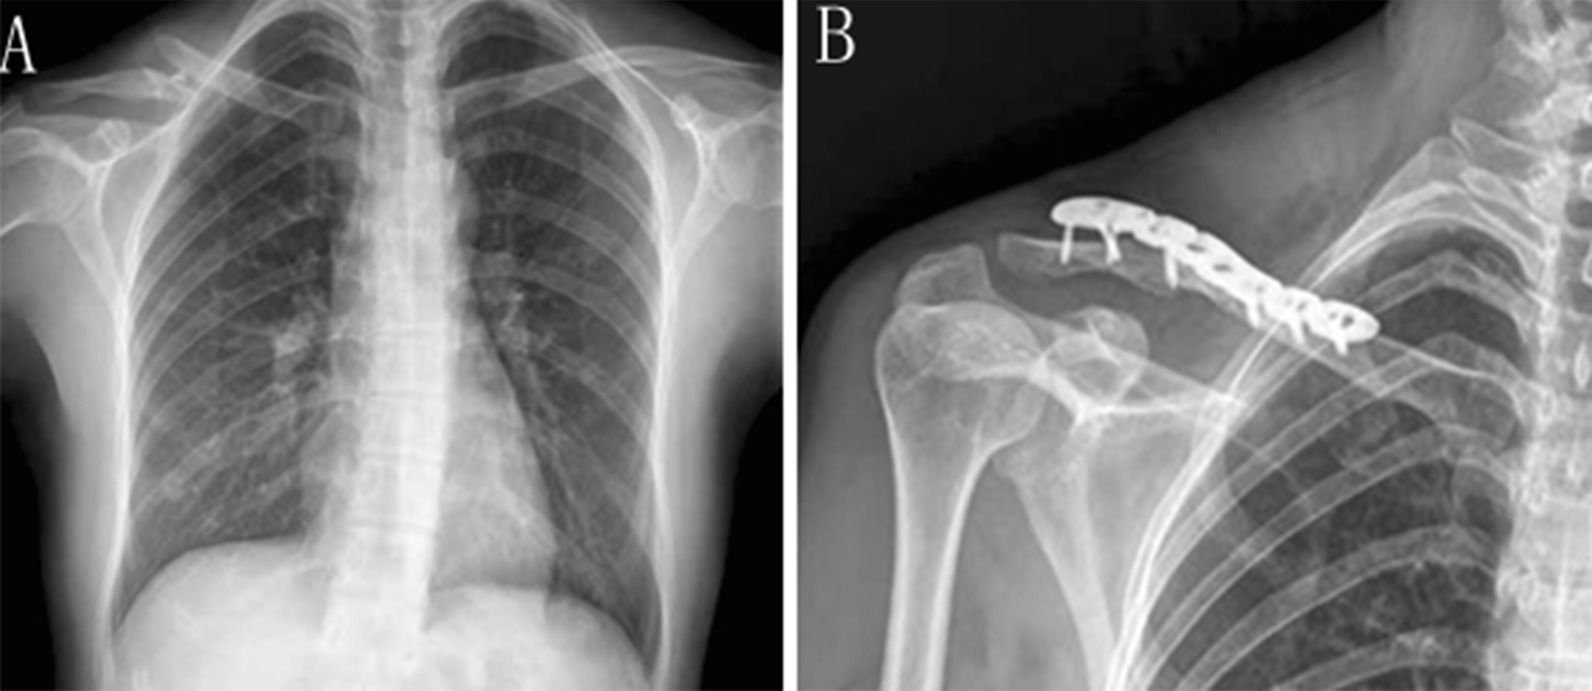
A Preoperative orthotopic chest radiograph. B Postoperative orthographic shoulder joint radiograph
